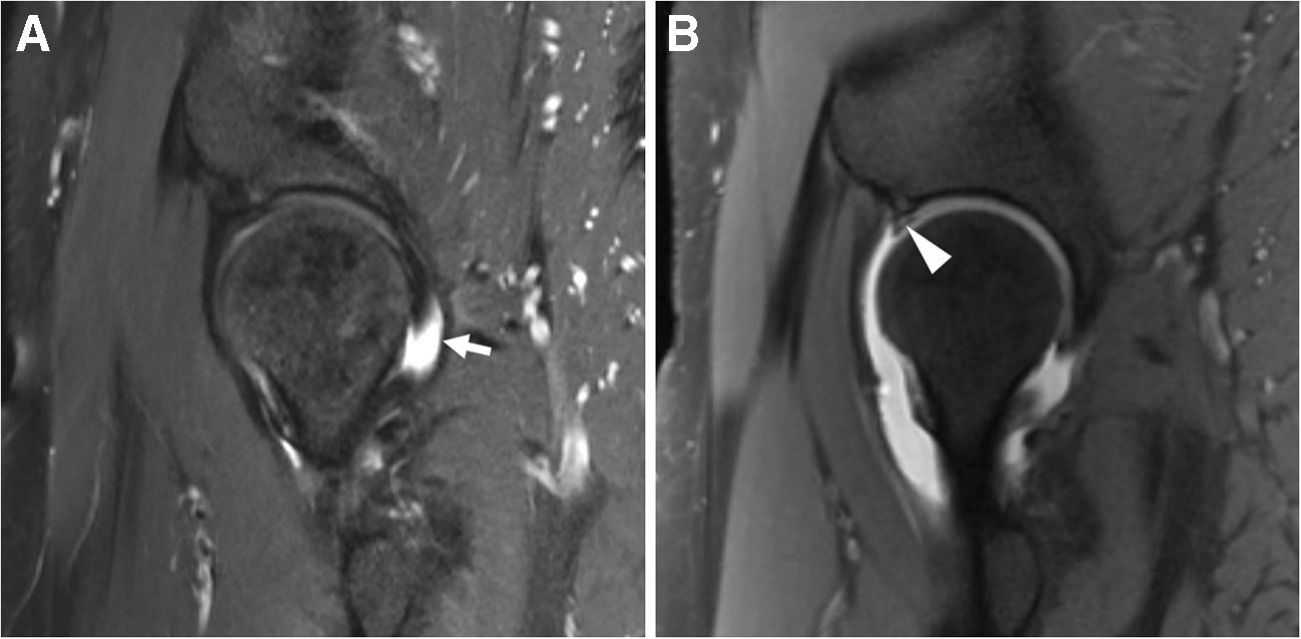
Labral tear. Forty-nine-year-old woman with hip pain. A Sagittal T2-weighted fat-suppressed conventional 3 T MR image shows a small, native joint effusion (arrow), but a labral tear is not clearly demonstrated. B Sagittal T1-weighted fat-suppressed 3 T MR arthrogram image shows contrast extending into the substance of the anterosuperior labrum (arrowhead), consistent with a tear which was subsequently confirmed at arthroscopy
